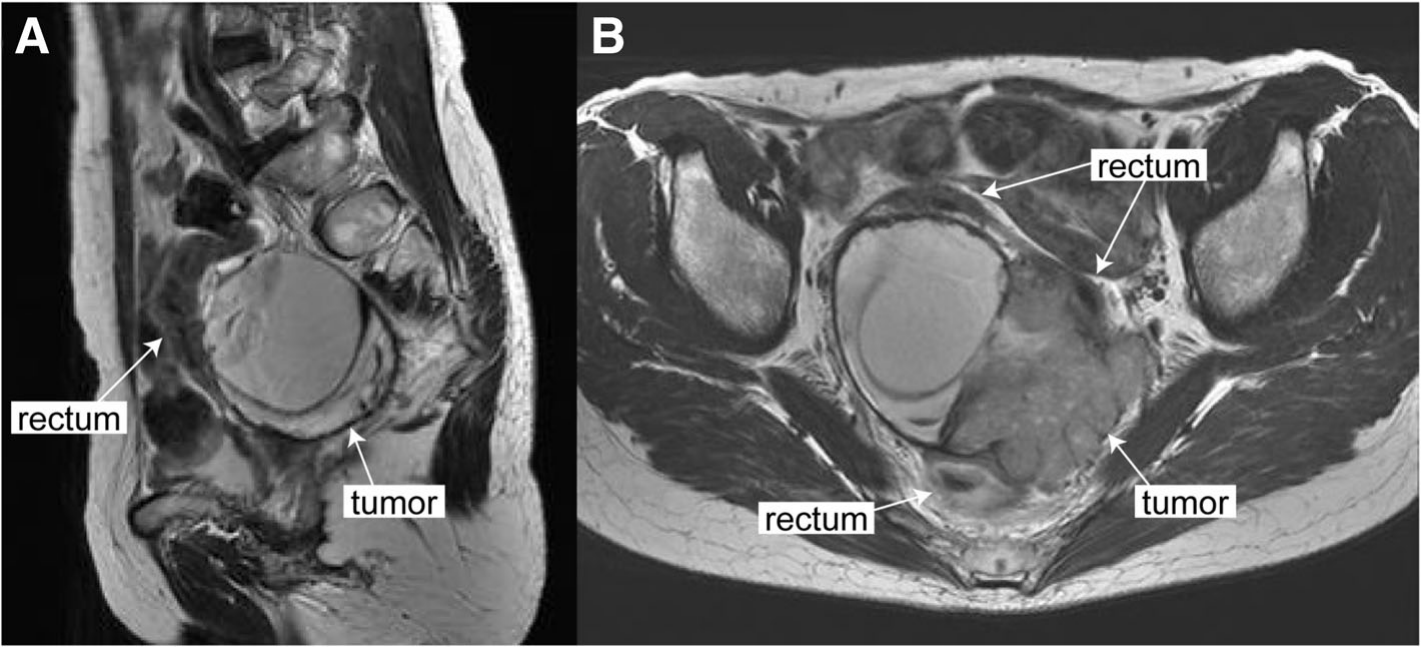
Preoperative T2-weighted magnetic resonance imaging showed the pelvic mass composed of cystic and solid parts in a axial and b sagittal views. Arrowheads indicate the tumor and rectum in each panel
